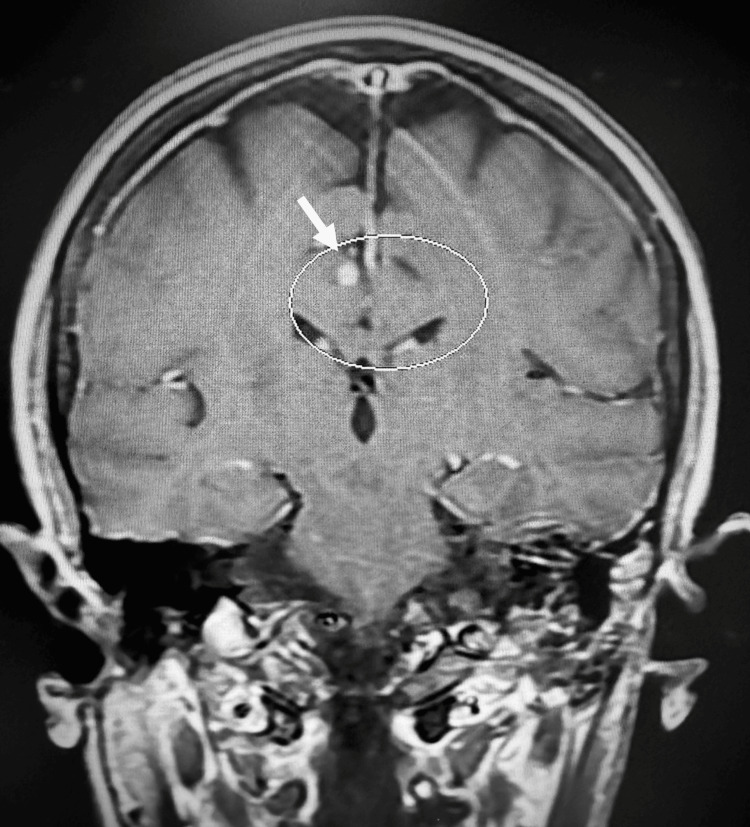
MRI of the head shows a new 3-mm enhancing lesion in the right frontoparietal parasagittal cortex.
